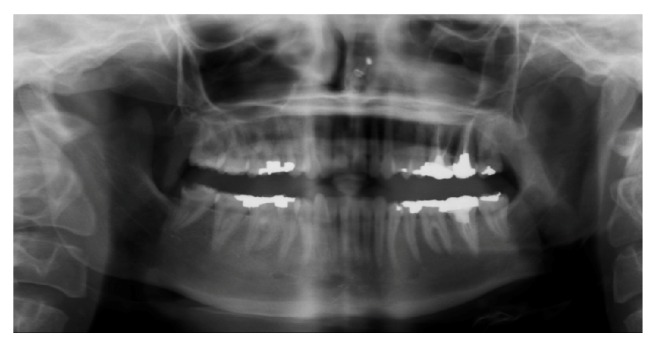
Panoramic radiograph of the patient's dental condition on day 15 of first hospitalization.
